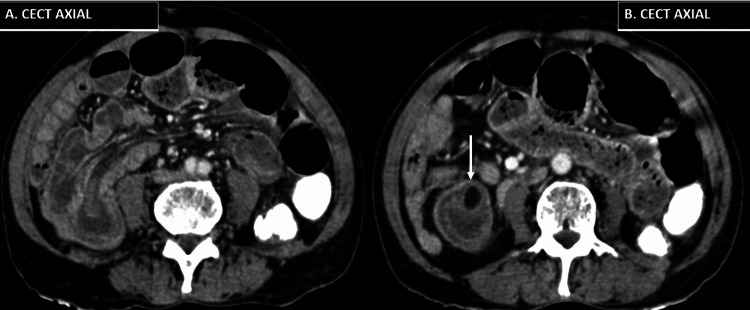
(A) Contrast-enhanced axial CT image showing bowel within bowel along with mesenteric fat and vessels, (B) Contrast-enhanced axial CT showing well-defined fat attenuation lipoma (white arrow) as the leading point of ileocolic intussusception.
